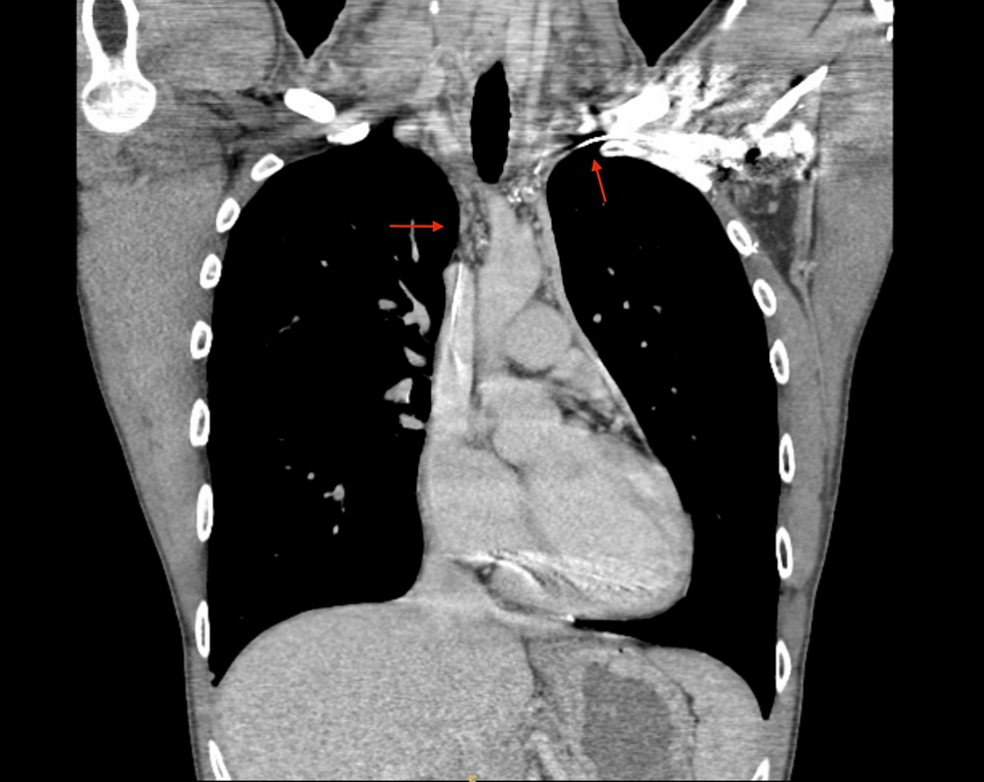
CT venogram of the chest The red arrow on the right depicts an occlusive thrombus of the right internal jugular vein with chronic total occlusion of the superior vena cava above the level of the azygos vein, along with occlusion of the right brachiocephalic vein. This also extends into chronic occlusions of the left brachiocephalic vein, which contains an automatic implantable cardioverter-defibrillator wire, indicated by the left red arrow.
